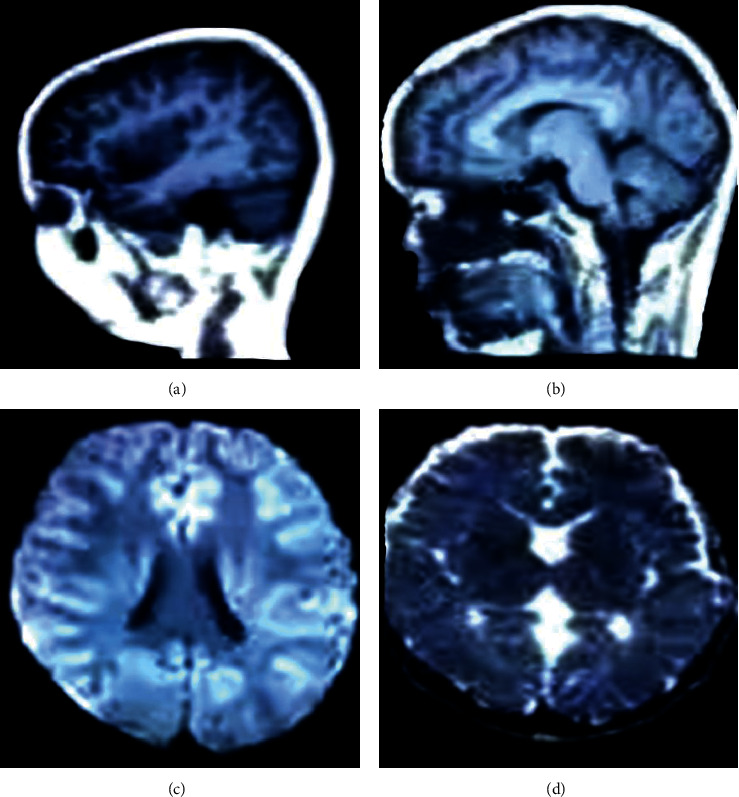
MRI results: (a) lateral ventricle, (b) cerebral aqueduct, (c) fourth ventricle, and (d) third ventricle.
